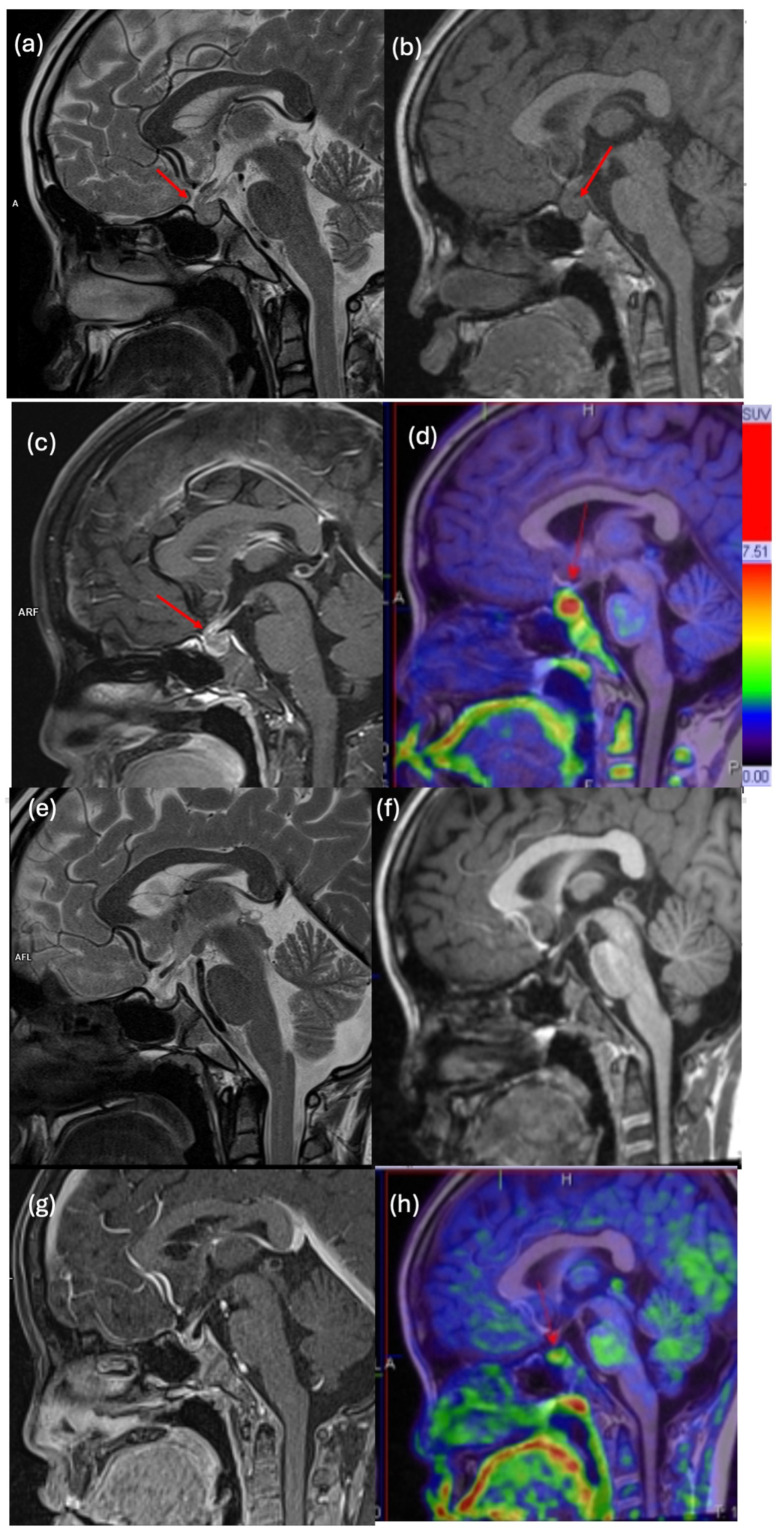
Suprasellar germinoma of a 14-year-old boy (No. 2). (a) T2-weighted sagittal scan showed pituitary stalk thickening with a prominent size of the pituitary gland. (b) Absent normal posterior pituitary T1-weighted bright spot on T1-weighted sagittal scan. (c) Enhancement on post-gadolinium T1-weighted scan and (d) strong C11-methionine tracer uptake noted within the pituitary gland and thickened pituitary stalk on PET-MRI [arrows]. (e) PET-MRI at 3 months post-treatment showed a significant interval decrease in size of the intrasellar mass with minimal residual pituitary stalk thickening on T2-weighted sagittal scan. (f) Absent normal posterior pituitary T1-weighted bright spot on T1-weighted sagittal scan. (g) Post-gadolinium T1-weighted scan. (h) Normalization of the MET tracer uptake in the pituitary gland without discernible MET uptake along the pituitary stalk on C11-methionine PET-MRI, indicating complete metabolic response [arrows]. There was no significant MET tracer uptake on further surveillance MET PET-MRI.
